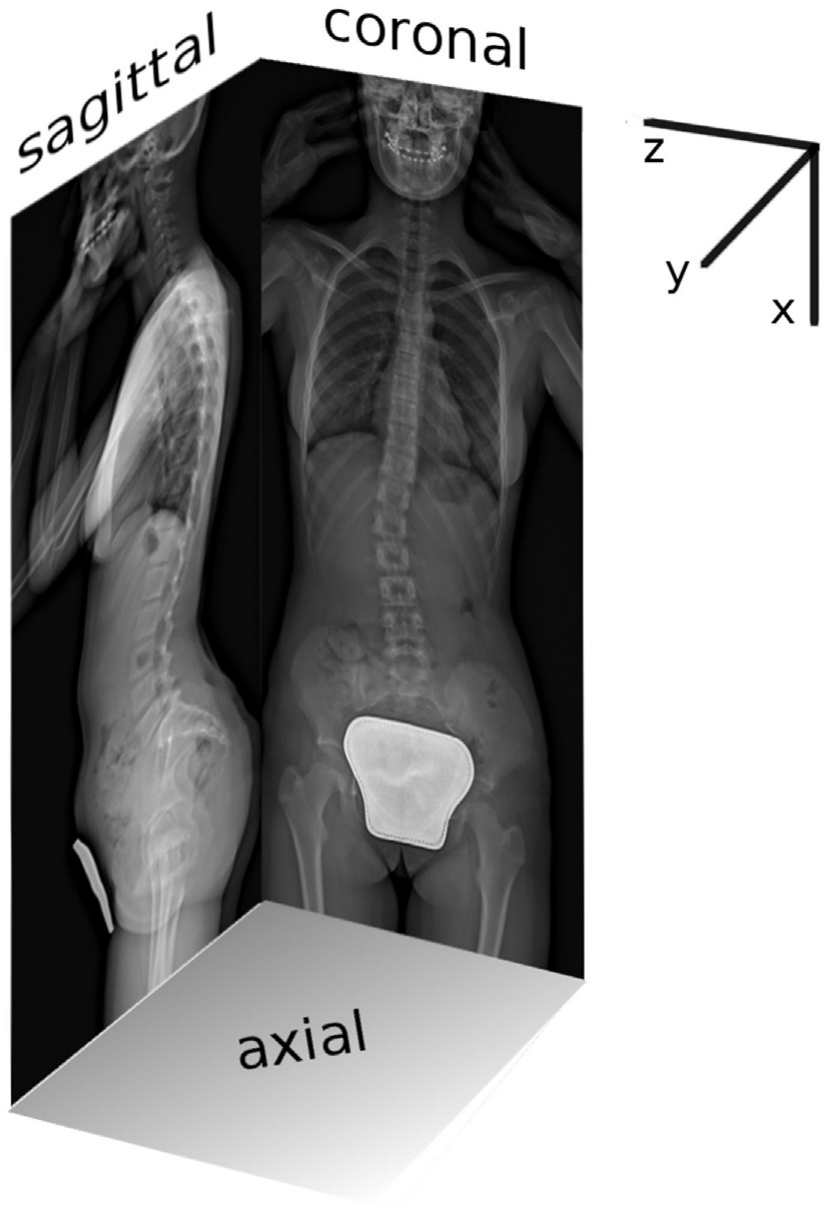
Coronal and sagittal planar radiographic images acquired with EOS system as simultaneous projection of the global reference 3D system {*x,y,z*}.
